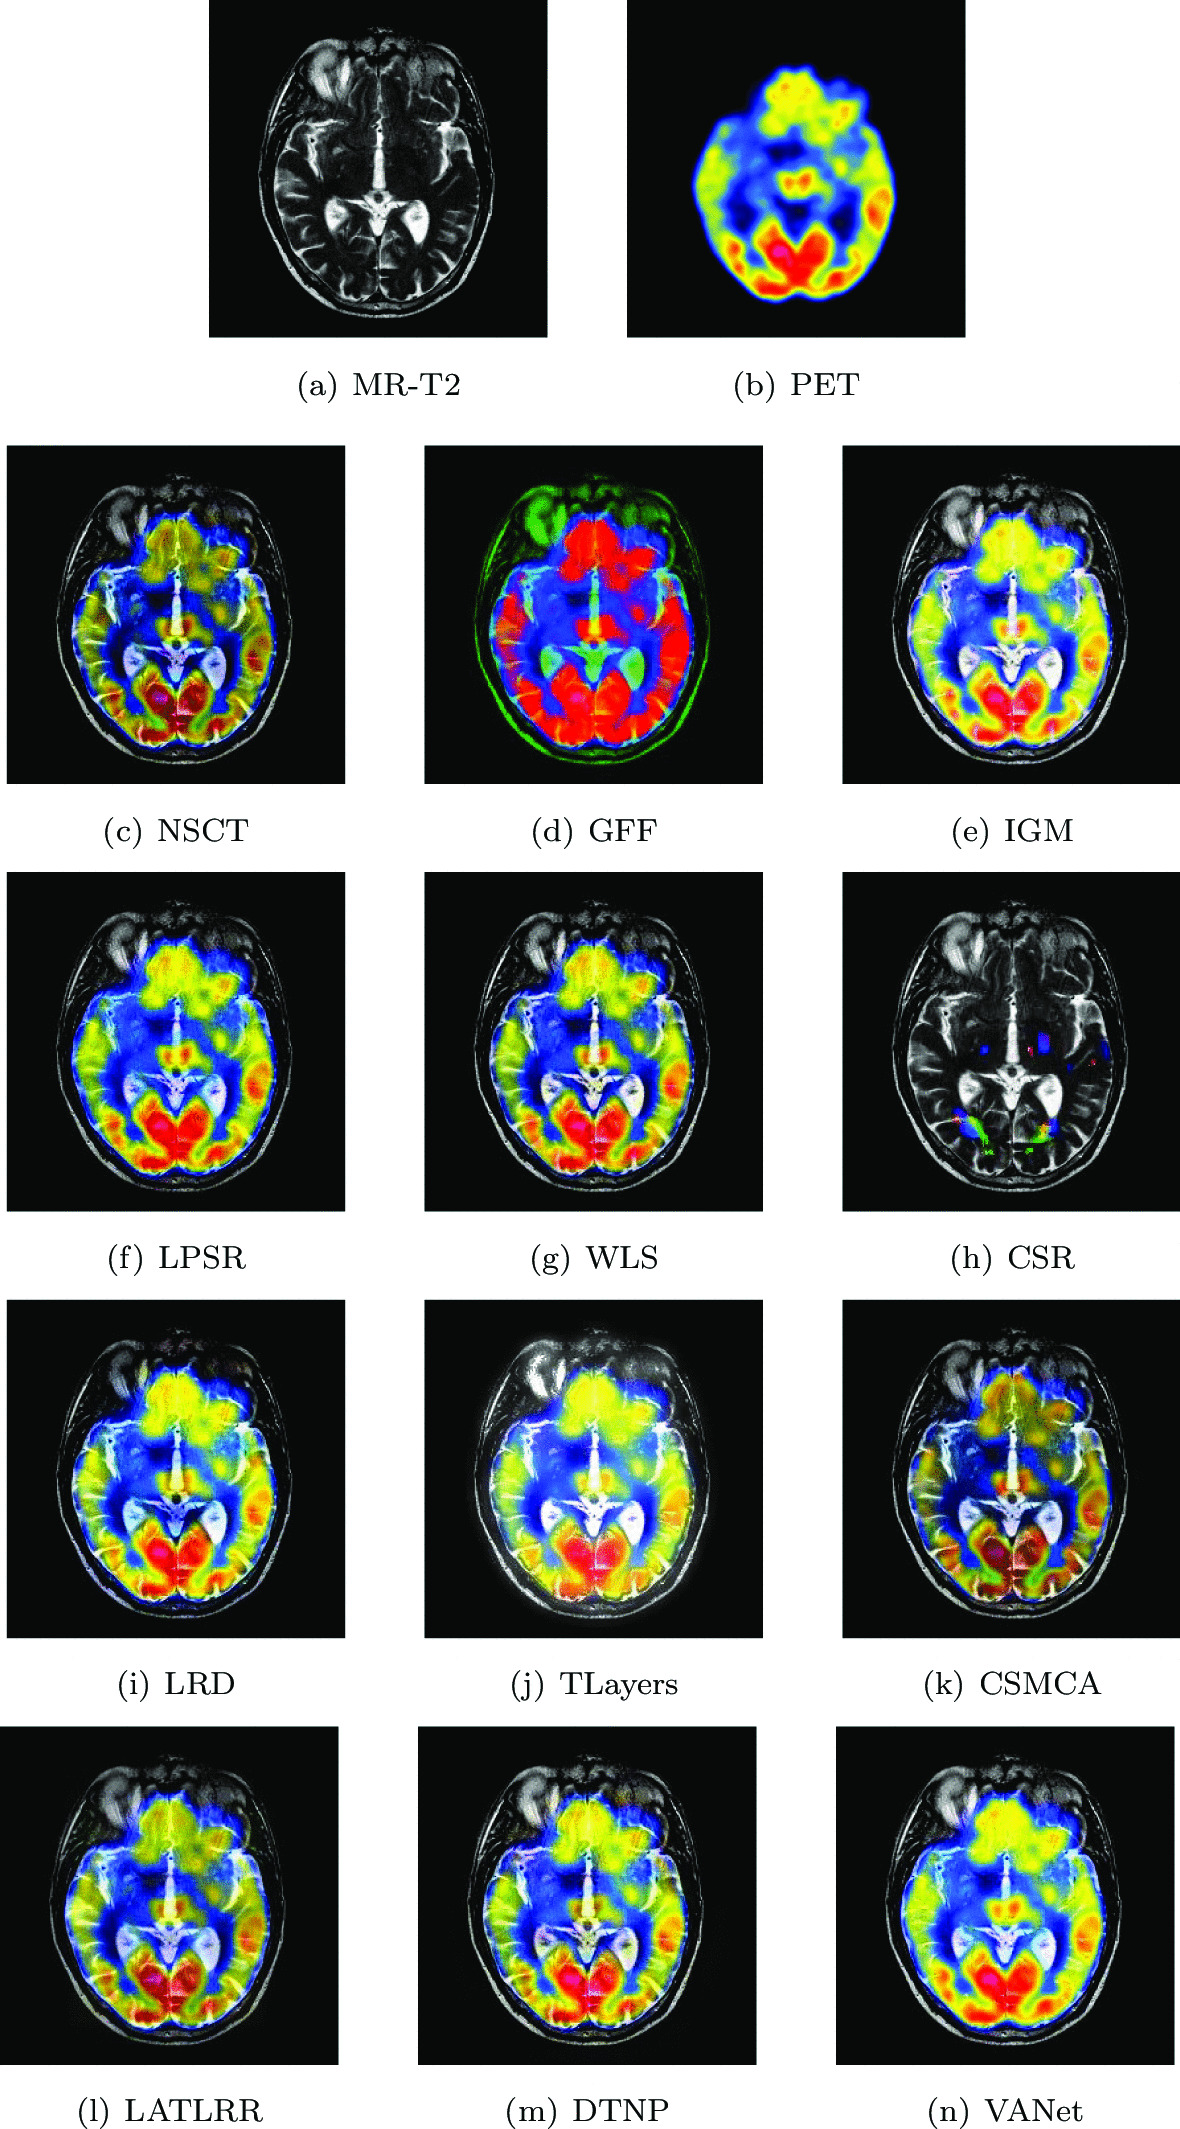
The first set of fused MRI-PET images from 12 methods on mild Alzheimer’s disease
